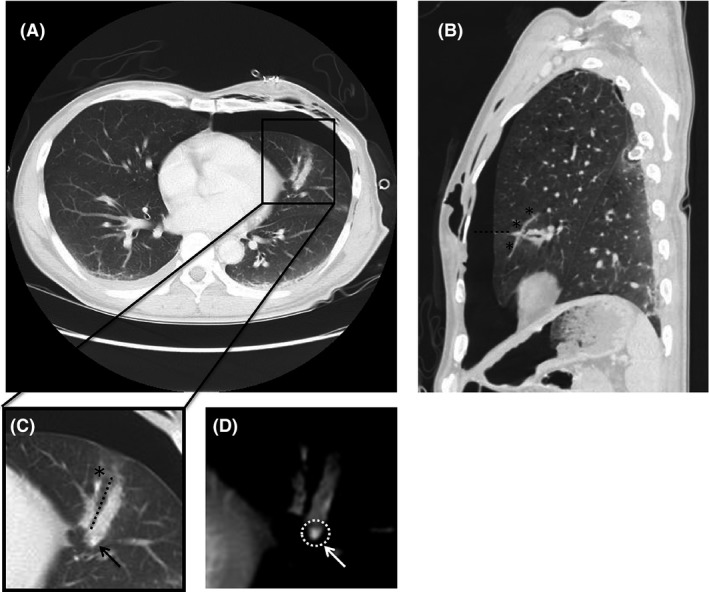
Axial (A) and sagittal (B) contrast‐enhanced computed tomography images of a 44‐year‐old man with self‐inflicted stab wounds to the anterior neck and left thorax. The images show the lung injury at the left lung lingular division (black square), and a pulmonary vein (black asterisk) around the lung injury (black dotted line). Magnified contrast‐enhanced computed tomography images of the arterial phase (C, D) show a pulmonary vein (black arrow, white arrow) and a pulmonary artery (black asterisk) near the consolidation of the lung injury (black dotted line).
